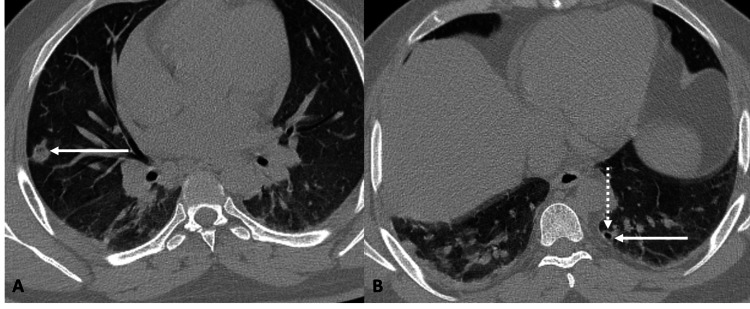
High-resolution computed tomography (HRCT) of the thorax A & B: Axial sections of the thorax in the lung window. Two nodules (solid white arrows), with one of them showing cavitation (dotted white arrow), are predominantly in the peripheral and subpleural region of bilateral lung fields.
